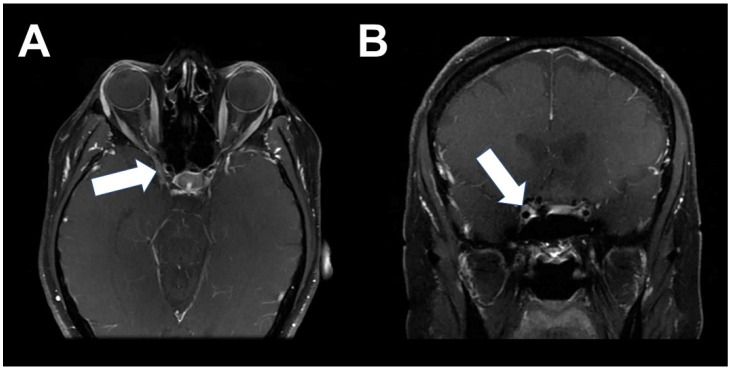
Post-contrast axial T1-weighted fat-saturated magnetic resonance images demonstrate an enhancing tubular structure (A,B, arrows), with increased posterior extension into the superolateral right cavernous sinus. It courses anteriorly through the right superior orbital fissure and bifurcates in the intraconal space at the orbital apex, before terminating along the lateral aspect of the right medial rectus and superior aspect of the right inferior rectus. Findings are consistent with underlying infiltrative orbital metastatic disease with optic nerve involvement in the setting of known invasive lobular carcinoma.
